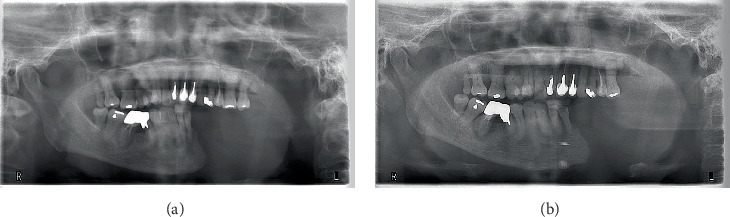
Orthopantomogram of the mandible. (a) Before secondary revision surgery. Hemimandibulectomy was performed between the left lower first and second teeth. (b) After secondary revision surgery. Two Mitek QuickAnchor Plus devices are visible at the margin of the mandibular resection. The mandibular position remains largely unchanged from the preoperative position, and no deterioration in occlusion is observed.
